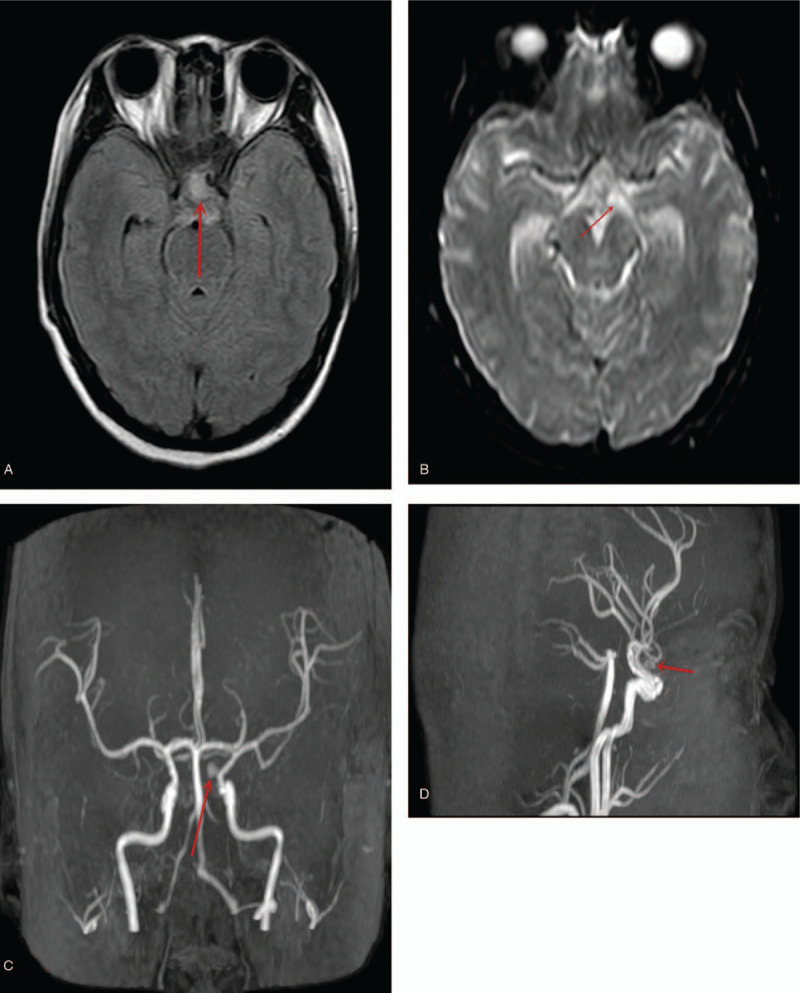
(A) Preoperative magnetic resonance imaging (MRI) showing high signal intensity in suprasellar cistern on FLAIR sequence. (B) Preoperative MRI showing high signal intensity was seen in the subarachnoid space on diffusion-weighted imaging sequence. (C and D) Magnetic resonance angiography images showing a dissected aneurysm in the ophthalmic segment of the left internal carotid artery.
